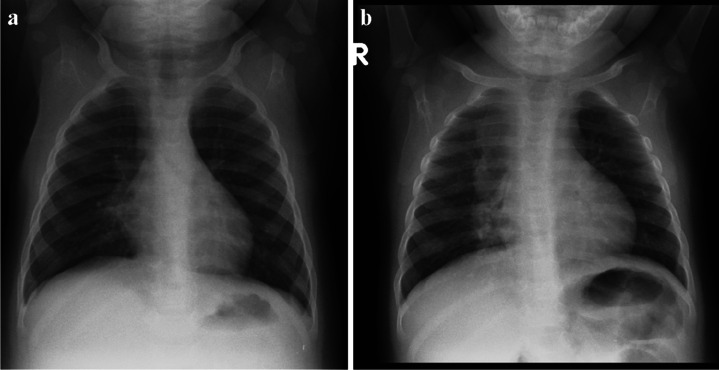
A 3-year-old boy newly diagnosed with human immunodeficiency virus infection and started on antiretroviral therapy. a Posteroanterior chest radiograph (CXR) on initiation of therapy shows no radiographic evidence of pulmonary tuberculosis (TB) and the patient was asymptomatic. b Repeat posteroanterior CXR 6 weeks later, on presentation with a cough, shows marked paratracheal, right hilar and subcarinal lymphadenopathy with splaying of the carina and attenuation of the bronchus intermedius and middle/lower lobe bronchi in keeping with TB. This is an example of unmasking TB immune reconstitution inflammatory syndrome
